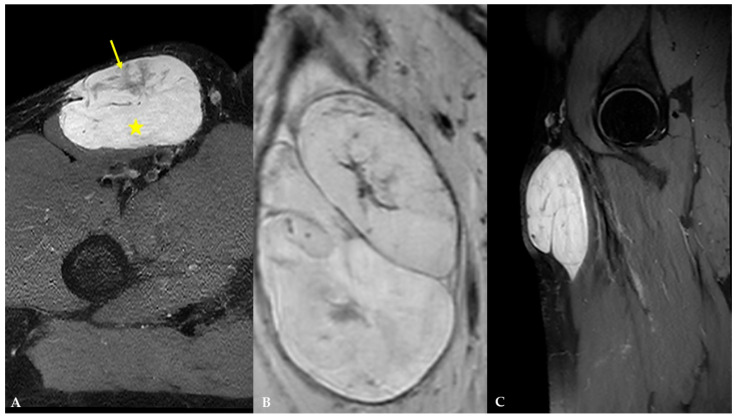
MRI features of MLS in the different planes: (A) axial plane, proton density (PD) fast spin echo (FSE) sequence with fat saturation demonstrating a hyperintense, lobulated, well-marginated, encapsulated mass with internal, irregular, low- to intermediate-signal-intensity foci and septa representing a mass with a high proportion of myxoid component (star) and low proportion of non-fatty non-myxoid component (arrow); (B) coronal plane; T2W FSE-MX 3D sequence; (C) sagittal plane, PD FSE sequence with fat saturation.
